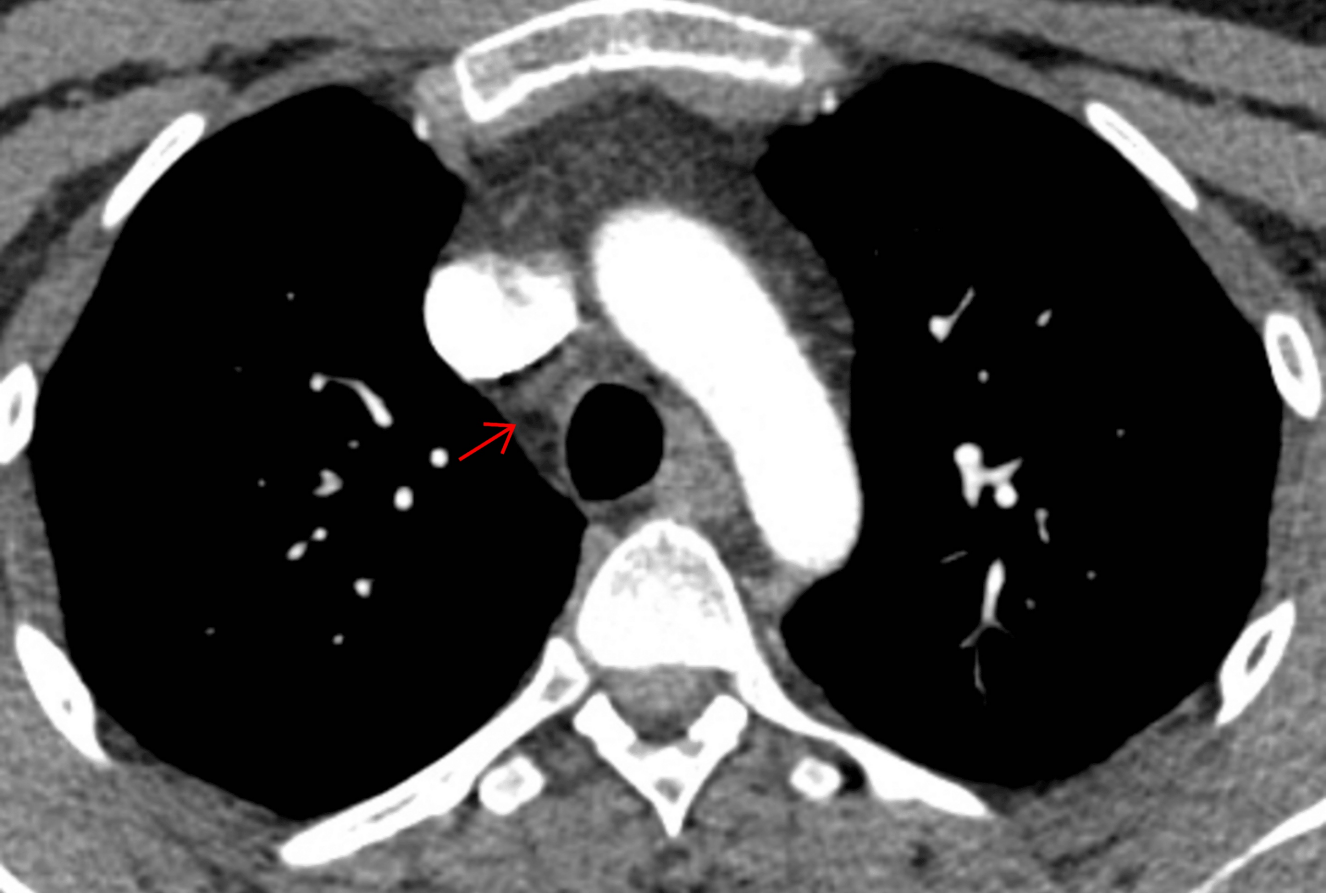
CT thorax showing complete resolution of right paratracheal lymph node CT: computed tomography
